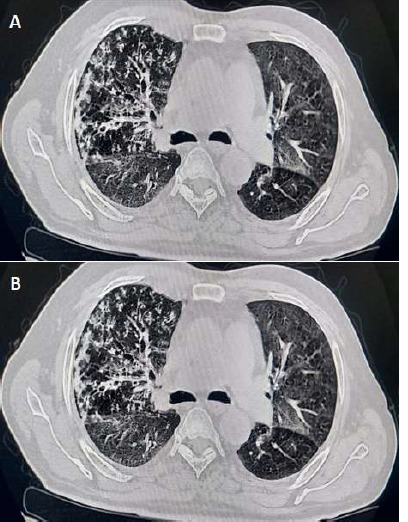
A) computerized tomography of the thorax prior to starting methotrexate therapy; B) high-resolution computed tomography scan of the thorax showing ground-glass opacities and interlobular septal thickening in the left lung and overlapping nodular and sub-pleural opacities in the right lung
